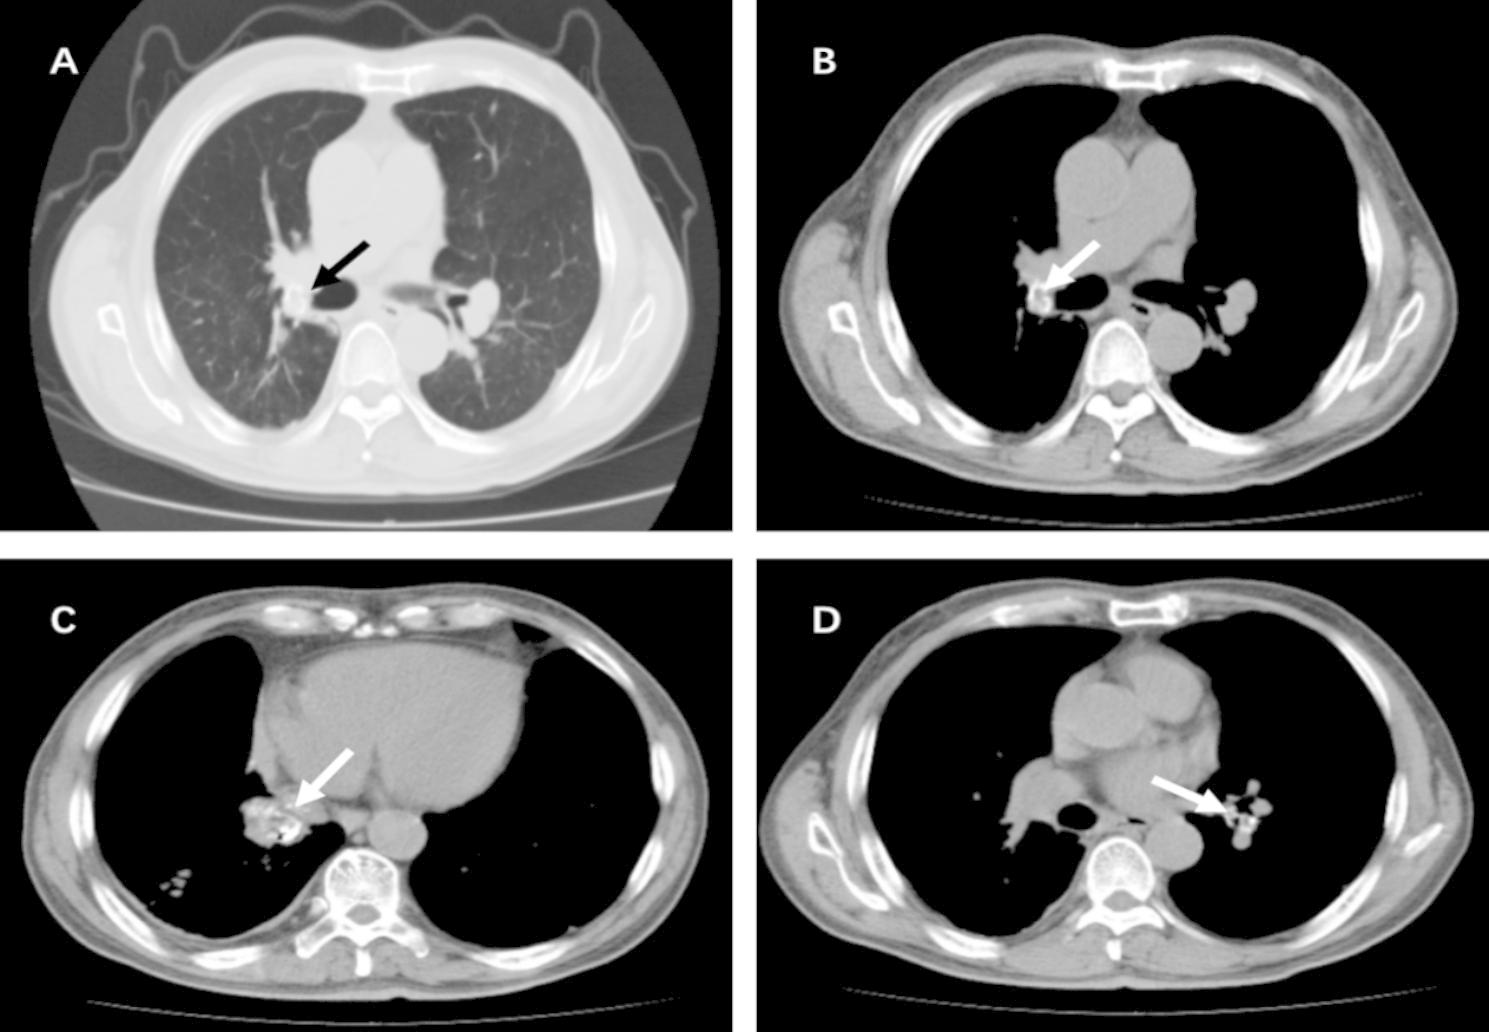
CT findings of multiple endobronchial masses. (A and B) Lung window and mediastinal chest CT scanning images showed an endobronchial mass (arrow) that obstructed the bronchi in the right upper lobe of the lung. (C) Mediastinal window showed a mass (arrow) that blocked the bronchi in the lower lobe of the right lung. (D) Mediastinal window showed obstruction (arrow) in the lower lobe of the left lung
